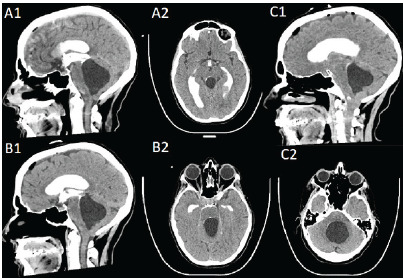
Computed tomography cisternogram. A: 10 minutes after contrast injection demonstrating obstructed flow into the 4th ventricle with some ventral spread of contrast. B: 30 minutes after contrast injection demonstrating further ventral descent of contrast. C: 105 minutes after contrast injection demonstrating ventrolateral spread of contrast to the obex of the 4th ventricle.
